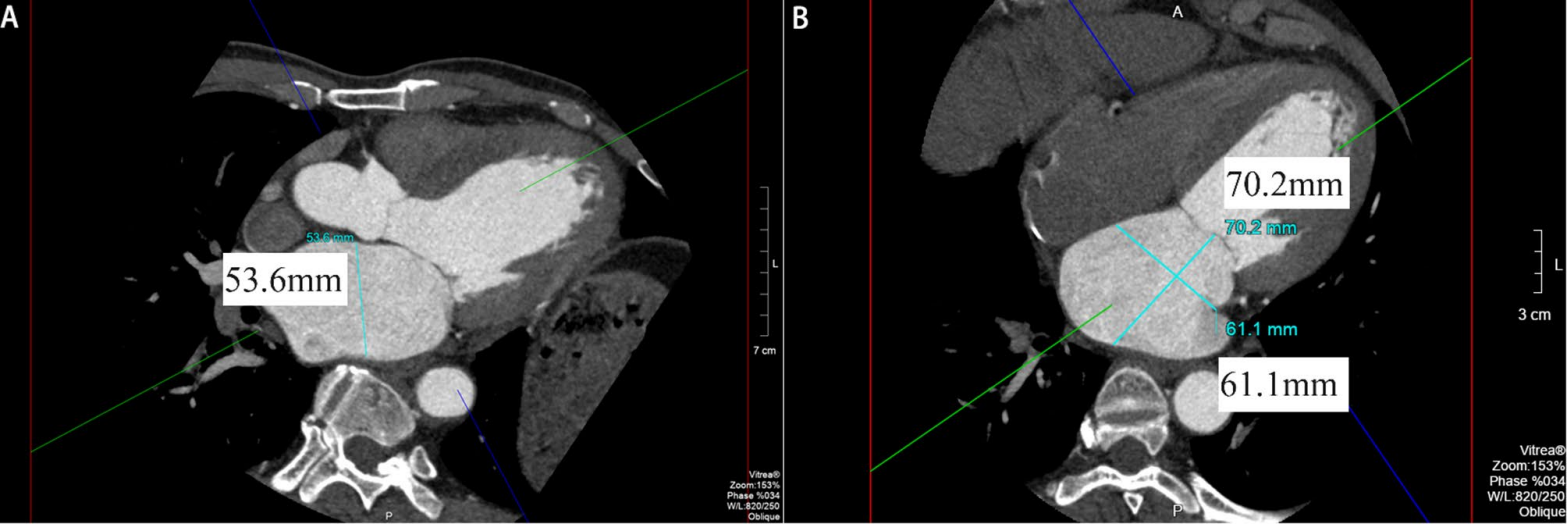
A: Echocardiography measurement of the LA anteroposterior diameter at the parasternal left ventricular long axis plane. B: Echocardiography measurement of the long diameter and transverse diameter of LA at the four-chamber plane.
